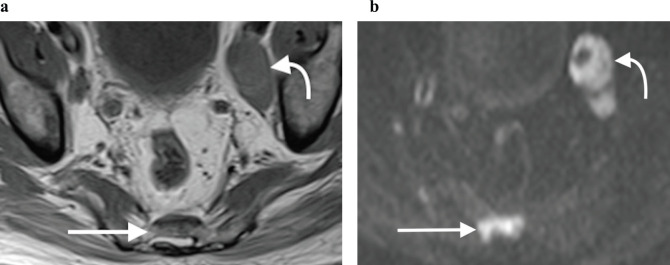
(a): Axial T1-weighted and (b): high b-value DWI showing obvious left pelvic lymphadenopathy (curved arrow) as well as a metastatic sacral bone lesion (straight arrow), which is subtle on the T1-weighted sequence but much more clearly depicted on the corresponding DWI sequence, due to marked restricted diffusion.
